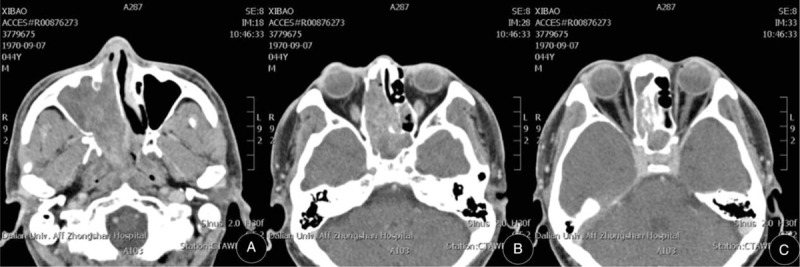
Paranasal sinus computed tomography scan shows paranasal sinus involvement (A), skull base erosion (B), orbit infiltration and intracranial involvement (C).
